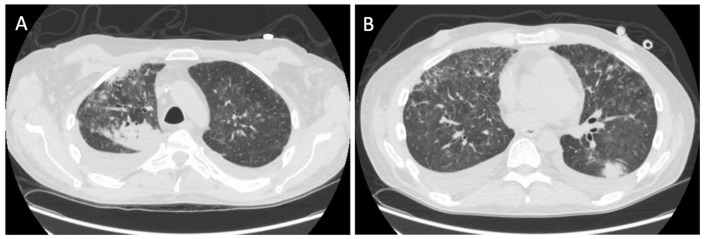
Computed tomography scan showing multifocal nodular opacities in the right upper lobe of lung (A) and the left lower lobe of lung (B).
